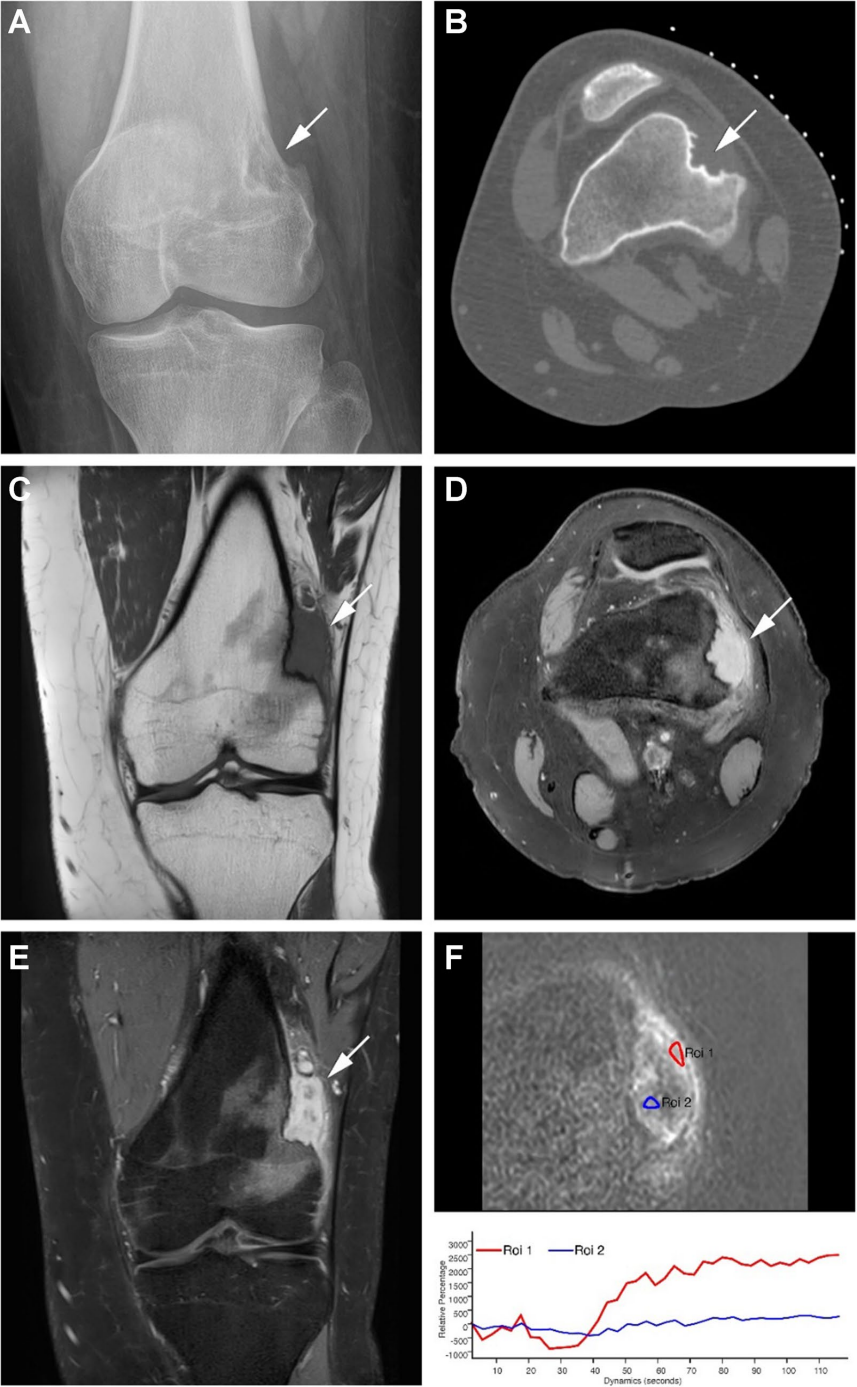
A: Distal femur (AP view): aggressive lytic lesion in the lateral distal femoral metaphysis eccentrically with marginal sclerosis medially but with apparent cortical destruction laterally (arrow). Figure 1B: CT scan (axial plane): cortical erosion with sclerotic underlying cortex (saucer-shaped defect) without matrix mineralization (arrow). Figure 1C: Coronal T1 weighted image (WI): lobulated and cortically based extrinsic erosion of bone and soft tissue extension (4.1 × 1.5 × 7.1 cm) in the distal femur metaphysis with an intermediate signal intensity on T1-weighted image (arrow). Figure 1D: Axial T2-weighted image with fat suppression (FS) and a high signal intensity: lobulated and cortically based lesion with extrinsic erosion of the distal femur metaphysis, with perifocal bone marrow edema as well as edema in the surrounding soft tissues (arrow). Figure 1E: Coronal T1 fat suppression (FS) contrast-enhanced weighted sequences: lesion with diffuse enhancement with small central areas lacking enhancement (arrow). Figure 1F: Dynamic contrast-enhanced magnetic resonance imaging (DCE-MRI) perfusion: rapid enhancement within 8 s after the artery, followed by a plateau phase; no washout was observed (type III enhancement curve) over all the tumor (RED region of interest, ROI). Several central areas in the tumor do not show contrast enhancement (type IV enhancement curve), (blue region of interest, ROI)
